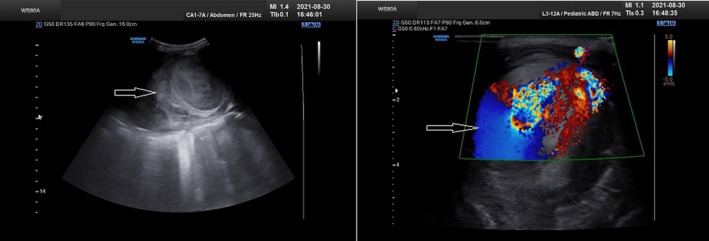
Ultrasound showed a large hematoma (arrow) posterior to the rectus muscle in the left lower quadrant. Superficial to the hematoma, there was an oval shape structure with turbulent flow in the Doppler study (to‐and‐fro pattern)
